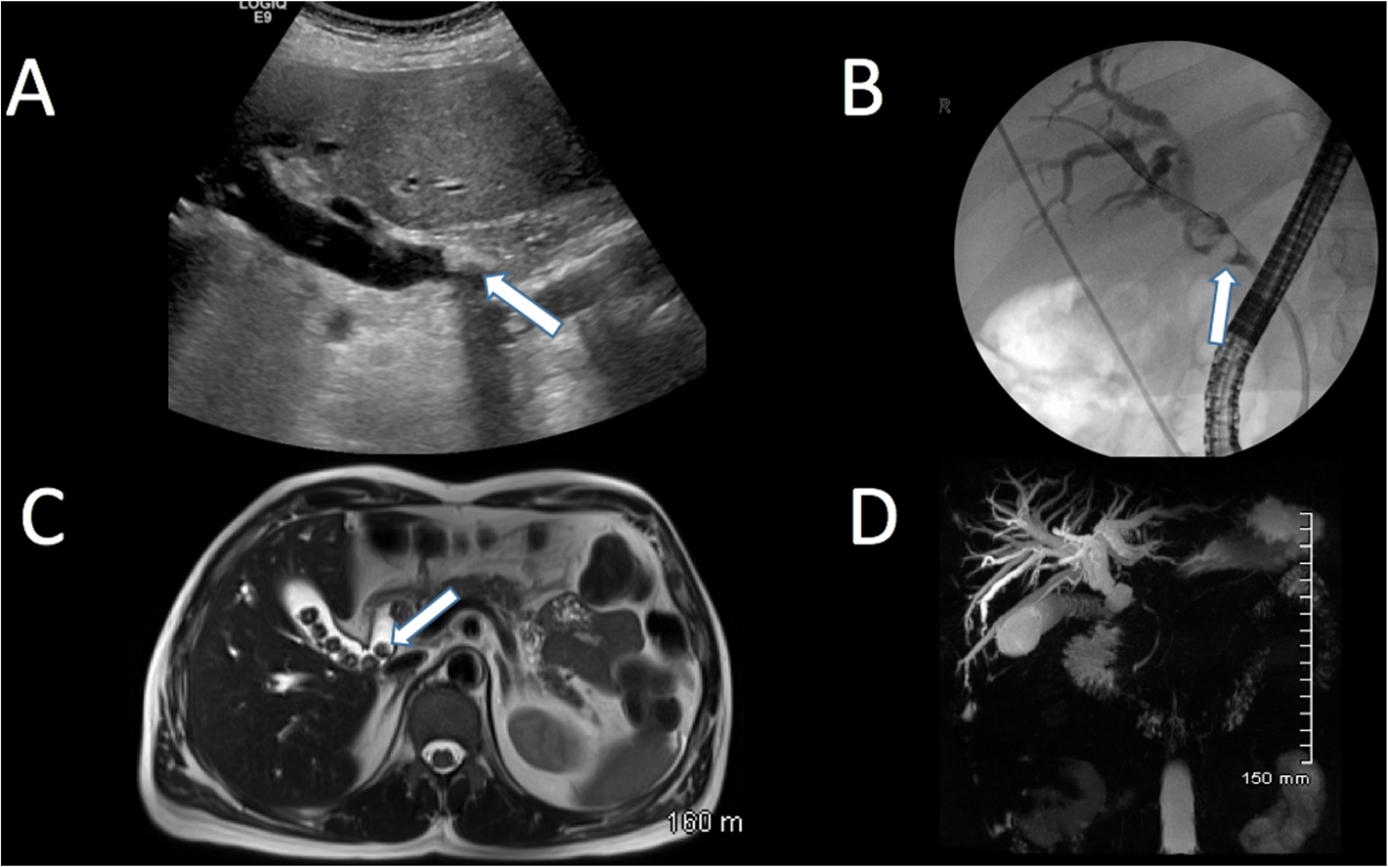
(a) Sagittal ultrasound, (b) fluoroscopic ERCP cholangiogram, (c) T2-weighted axial MRI and (d) coronal MIP MRI of the biliary tree. These images demonstrate multiple filling defects (arrows) within the common bile duct with associated biliary duct dilatation consistent with obstructing choledocholithiasis
